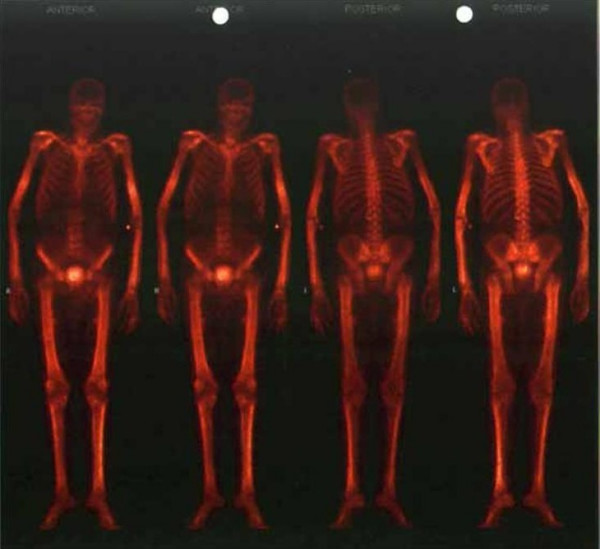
Skeletal scintigraphy showing diffusely increased uptake and the absent kidney sign (a super scan).
